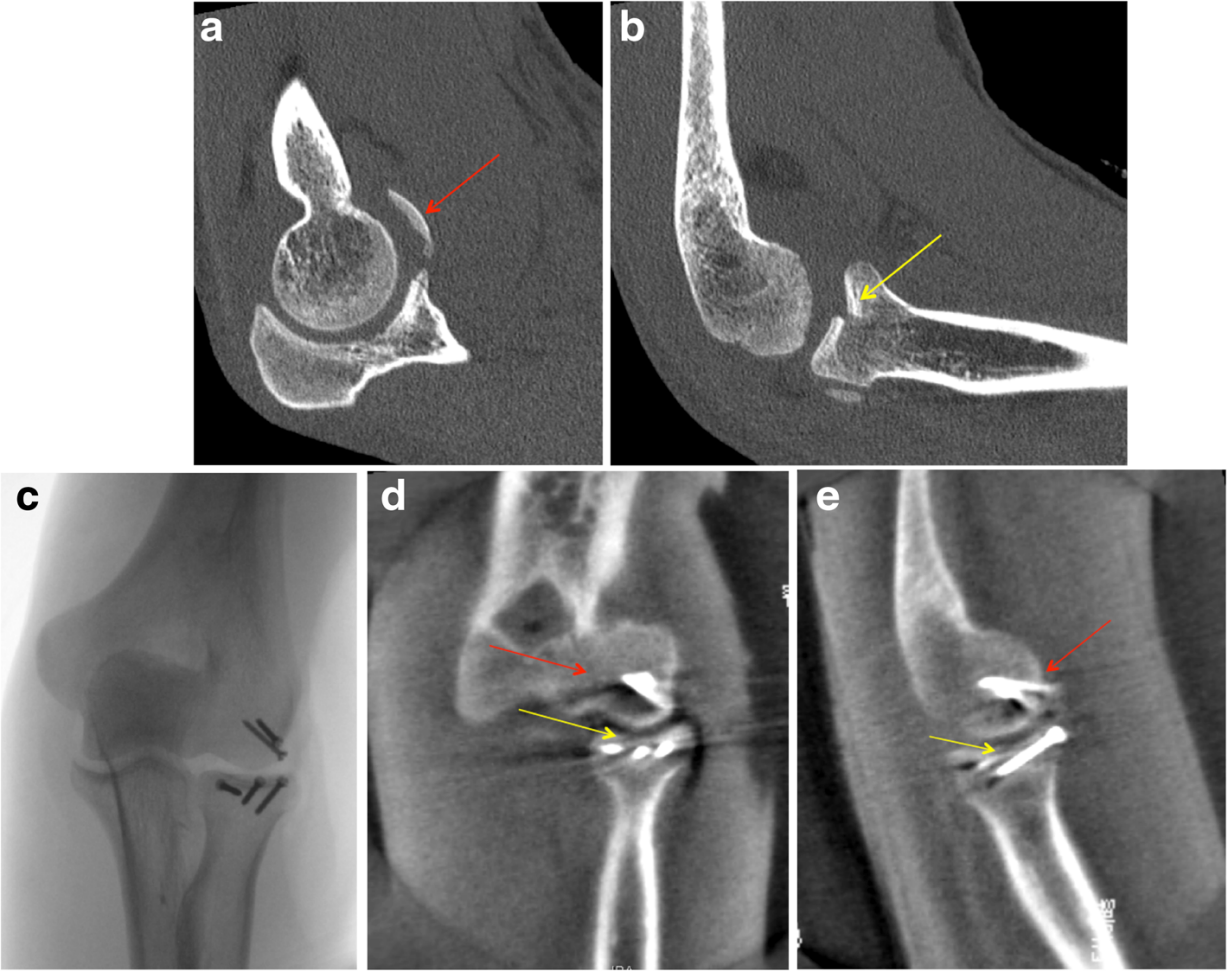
a, b Pre-operative computed tomography showing a complex elbow injury with a flake fracture (2x3cm, *red arrow*) of the capitellum and a radial head fracture type II according to Mason with a step of >2 mm in the joint surface (*yellow arrow*). c Intraoperative 2D fluoroscopy images after open reduction and fracture fixation with screws. d, e The fracture reduction of the capitellum could not be visualized intraoperatively. The intraoperative sagittal and coronal multi-planar-reconstructions of the 3D scan confirmed anatomical reduction of the capitellum (*red arrow*) as well as the radial head fracture (*yellow arrow*)
